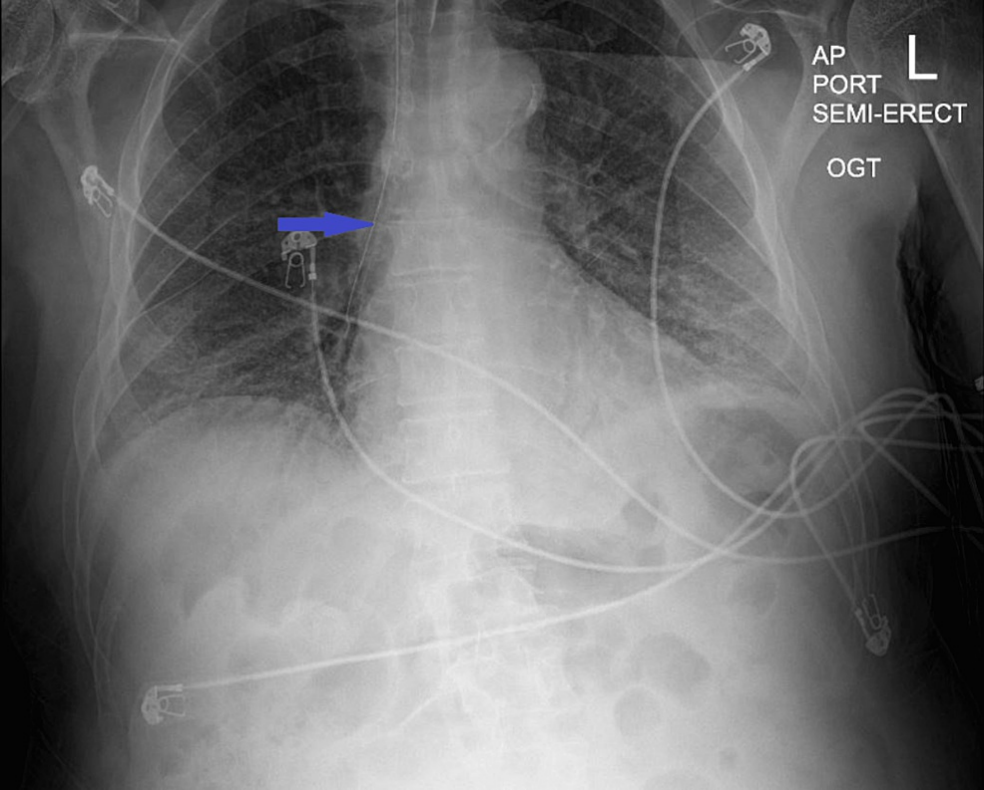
Antero-posterior X-ray showing pneumomediastinum with an orogastric tube in-situ.
